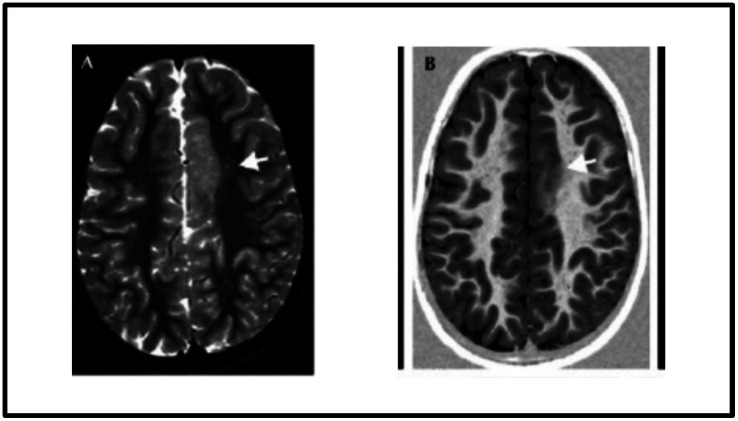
MRI images of FCD type II.
